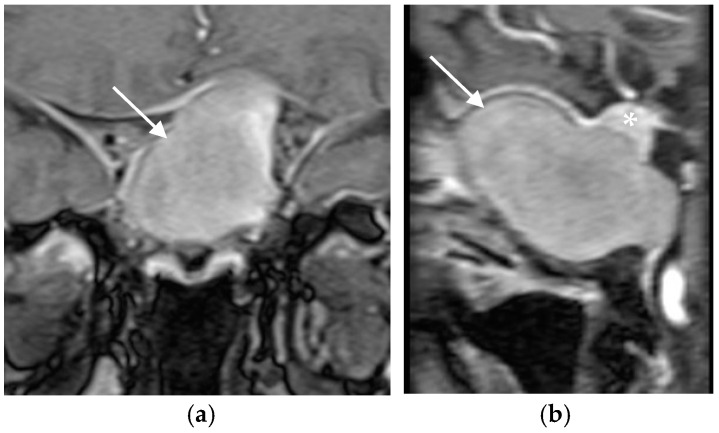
(a) Coronal and (b) sagital MRI scans of the sellar region before the transphenoidal operation showing giant hyperintense (on T_1_-weighted sequences) lesion in the sellar region (white arrows), with a thin rim of enhancement propagating in all directions (supra-, infra-, retro- and pre-sellar). The pituitary gland was displaced superiorly (asterix).
